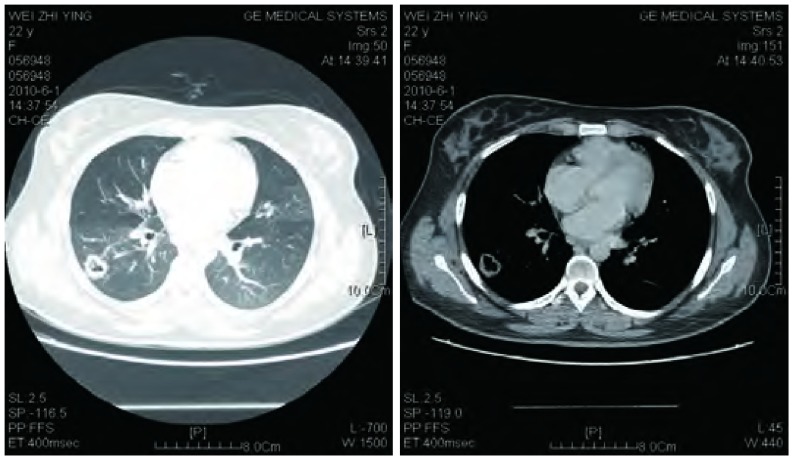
右肺下叶背段空洞性病变（2. 3 cm×1. 8 cm×2. 4 cm）（2010.06.01） The cavitation (2.3 cm×1.8 cm×2.4 cm) in apical segment of right lower lobe was no any alteration compared with 4 months ago CT finding (Jun 01, 2010)
